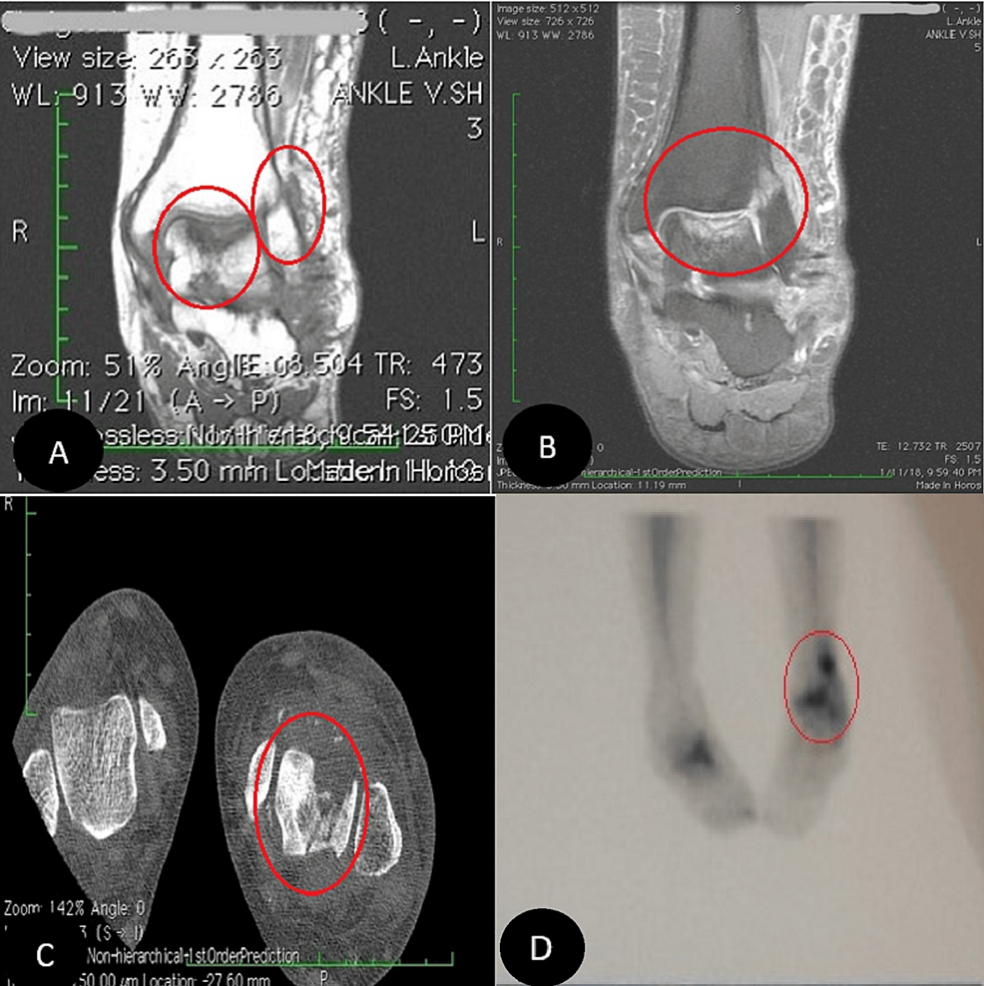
MRI, CT scan, and technetium-99m (Tc-99m) bone scan Coronal MRI confirmed avascular necrosis of the talar dome with subchondral fracture (A and B, respectively). CT scan (C) and Tc-99 bone scan (D) images also revealed the pathologies.
